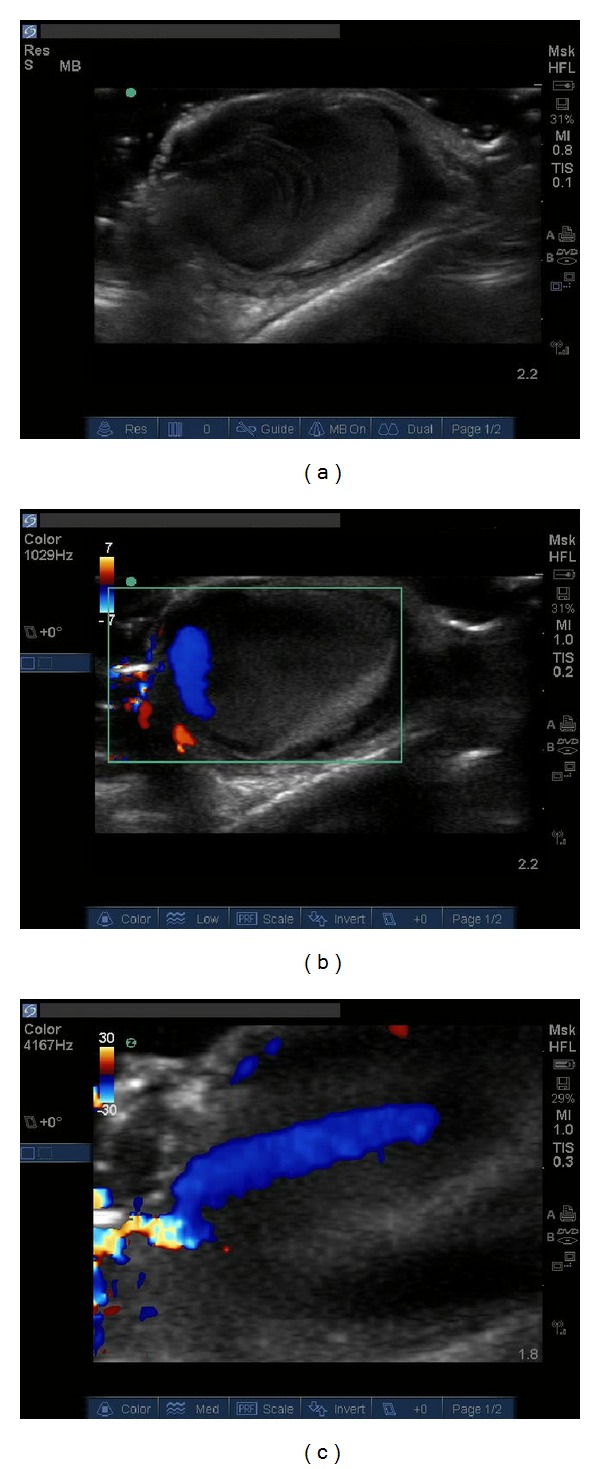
Ultrasound images of patient's pseudoaneurysm. (a) B-mode ultrasonography shows a fluctuant mass with internal echoes similar to an abscess. Dynamic images would reveal wave-like flow through the mass suggestive of communication with a vessel lumen. (b) Color Doppler ultrasonography confirms vascular communication and pulsatile flow. (c) Color Doppler ultrasonography zoomed in on the pseudoaneurysm neck further confirms communication of pseudoaneurysm with an arterial lumen (see Supplementary material which is available online at http://dx.doi.org/10.1155/2014/647175).
